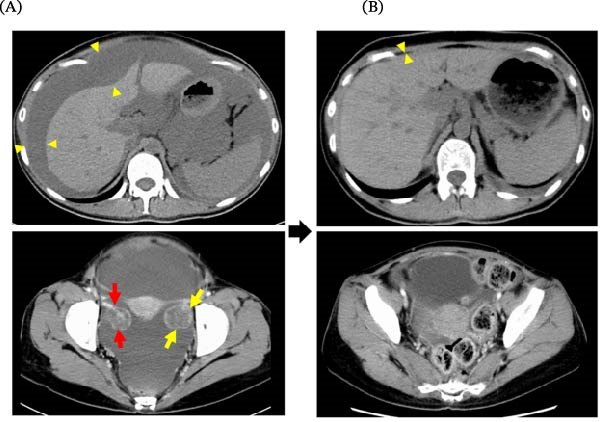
Perioperative computed tomography images of the abdomen and pelvis in Case 6. (A) The findings 2 weeks before surgery. (B) The findings 2 months after surgery. The yellow arrowhead indicates a marked reduction in perihepatic ascites. The yellow arrow indicates the enlarged left ovary, and the red arrow indicates the enlarged right ovary.
